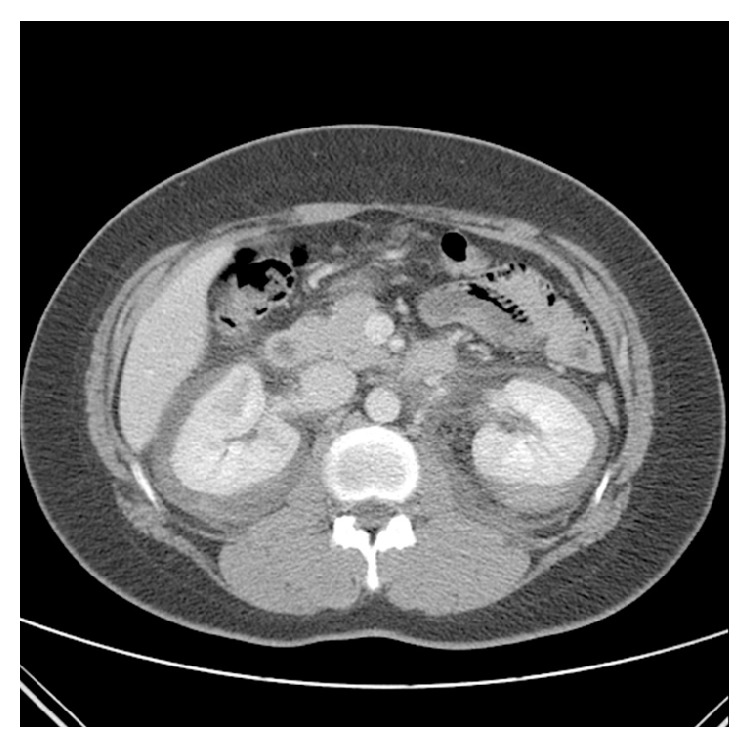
Contrast enhanced CT on the day of admission. CT revealed nonspecific spontaneous bilateral perirenal hemorrhage and delayed enhancing lesion of renal medulla.
